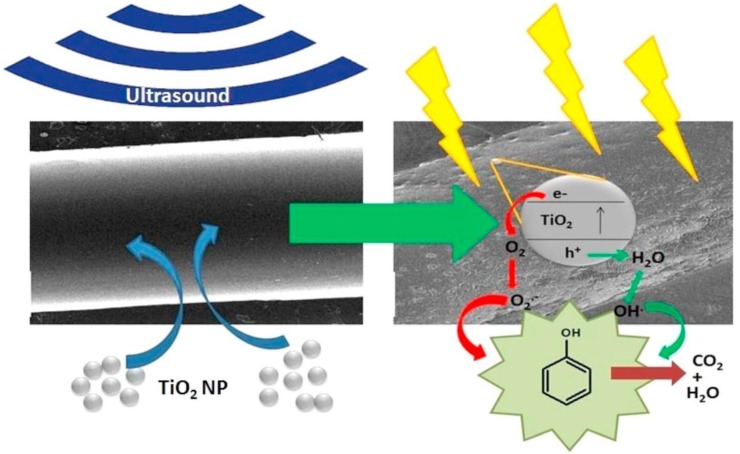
Photocatalytic phenol degradation in ultrasound (TiO_2_)-deposited FEP microtube. Reprinted from [93] with permission of Elsevier.
